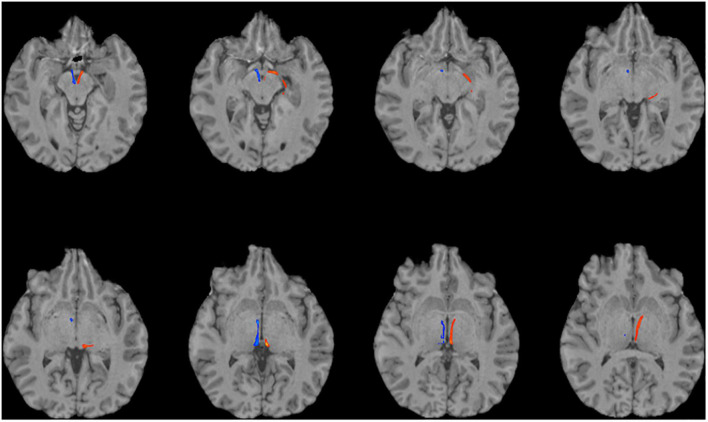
Using multi-shell “clinical grade” dMRI data to reconstruct the afferent and efferent tracts of the LHb. Axial T1 MR images with superimposed streamline density maps of LHb fiber tracts are shown for a representative subject. Left hemisphere streamlines are colored in red and right hemisphere streamlines are colored in blue.
